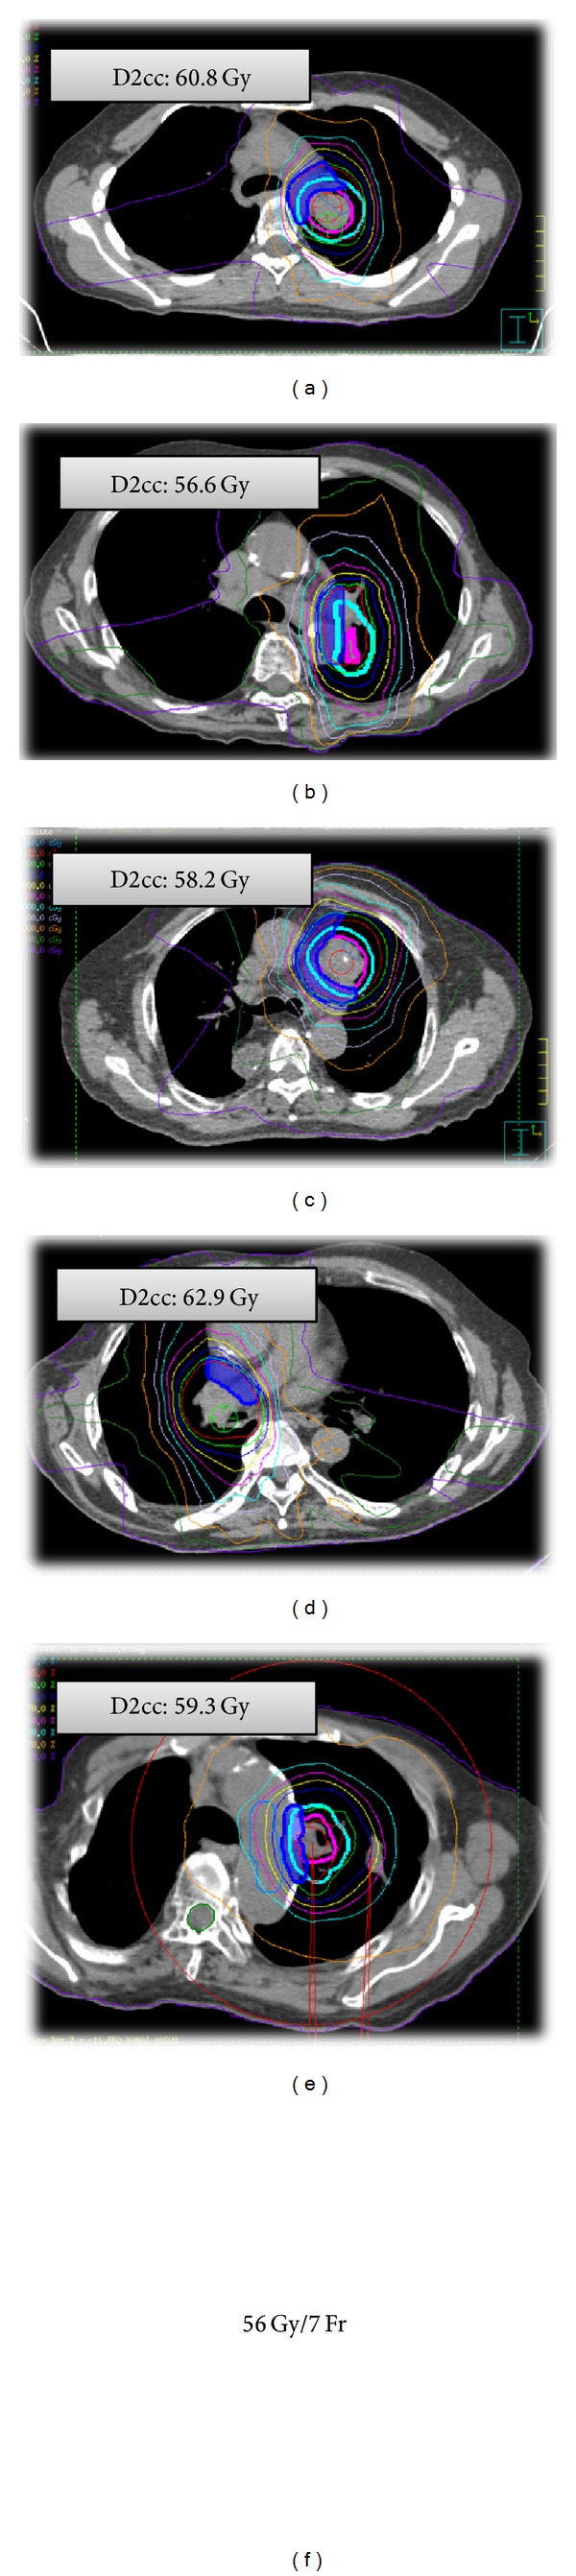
CT images showing dose distributions of the patients with top five cases of the minimum doses in the most irradiated 2 cc of the mediastinal structures (D2cc). Cyan, internal target volume (ITV); pink, planning target volume (PTV).
